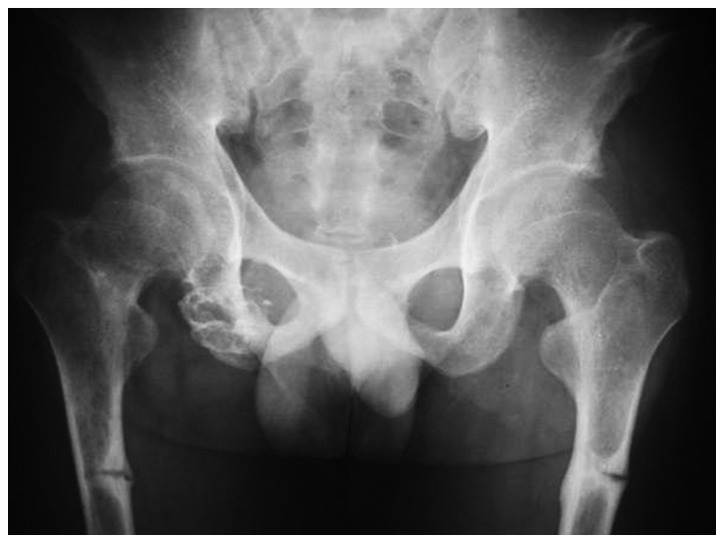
Case two: Plain radiograph showing a bony destructive lesion, with calcification in the right ischium and ununited fractures in the shafts of the bilateral femora (Looser’s zones).
